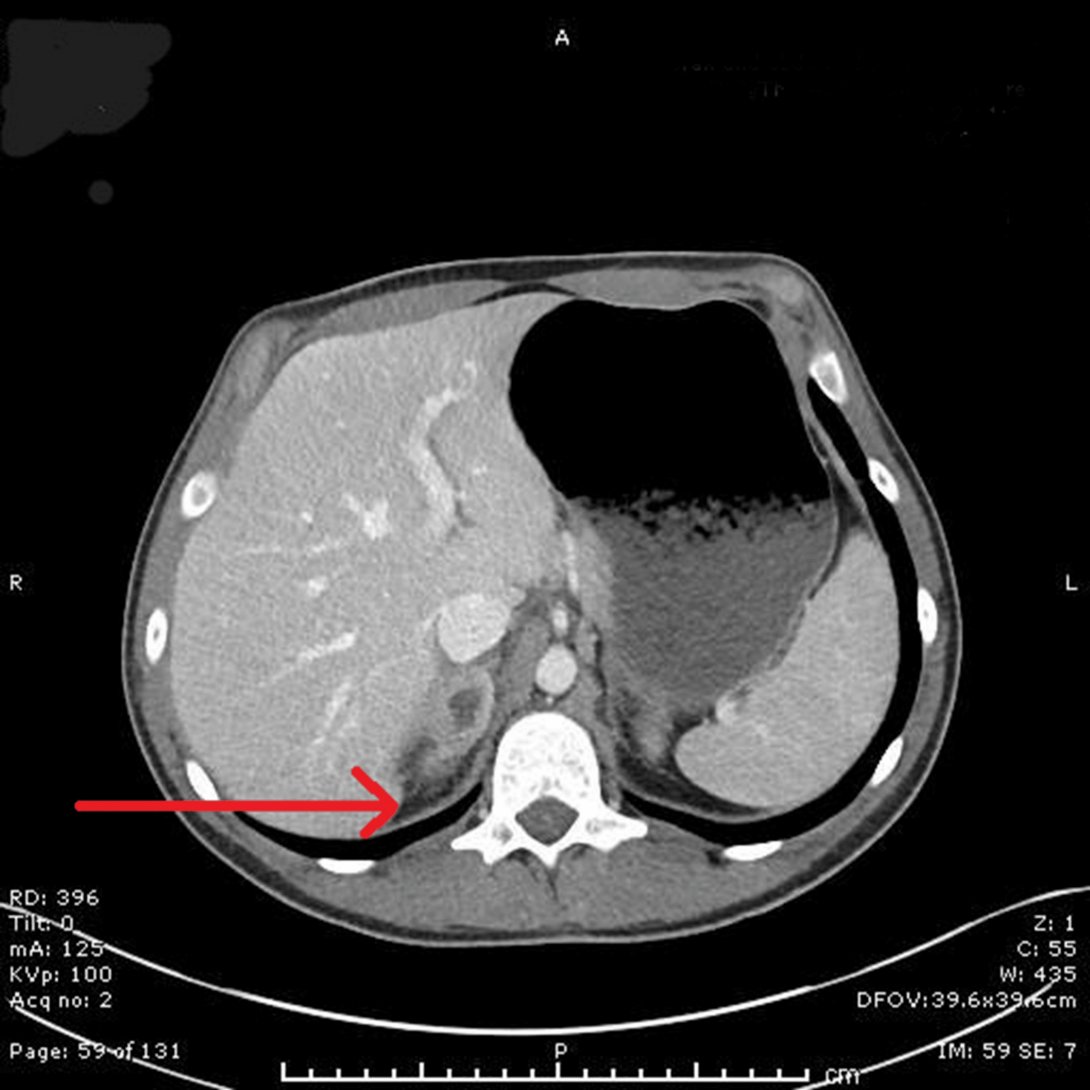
Contrast-enhanced CT (Day 38): Red arrow indicates reduced volume of right adrenal abscess; no evidence of recurrent left adrenal abscess
